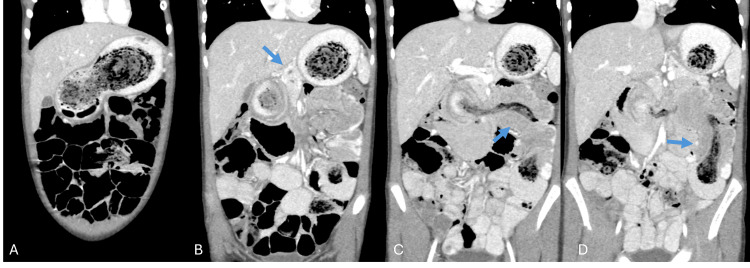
Sequential contrast-enhanced CT images of the abdomen and pelvis demonstrating a large gastric trichobezoar with proximal small bowel extension consistent with Rapunzel syndrome. (A) The coronal image shows a large intragastric trichobezoar. (B) The coronal image demonstrates mass effect on the pancreas with associated pancreatic duct dilation (blue arrow). (C-D) The coronal images demonstrate extension of the trichobezoar into the small bowel complicated by jejunojejunal intussusception (blue arrows). Additional distal extension of the trichobezoar was present but is not shown.
